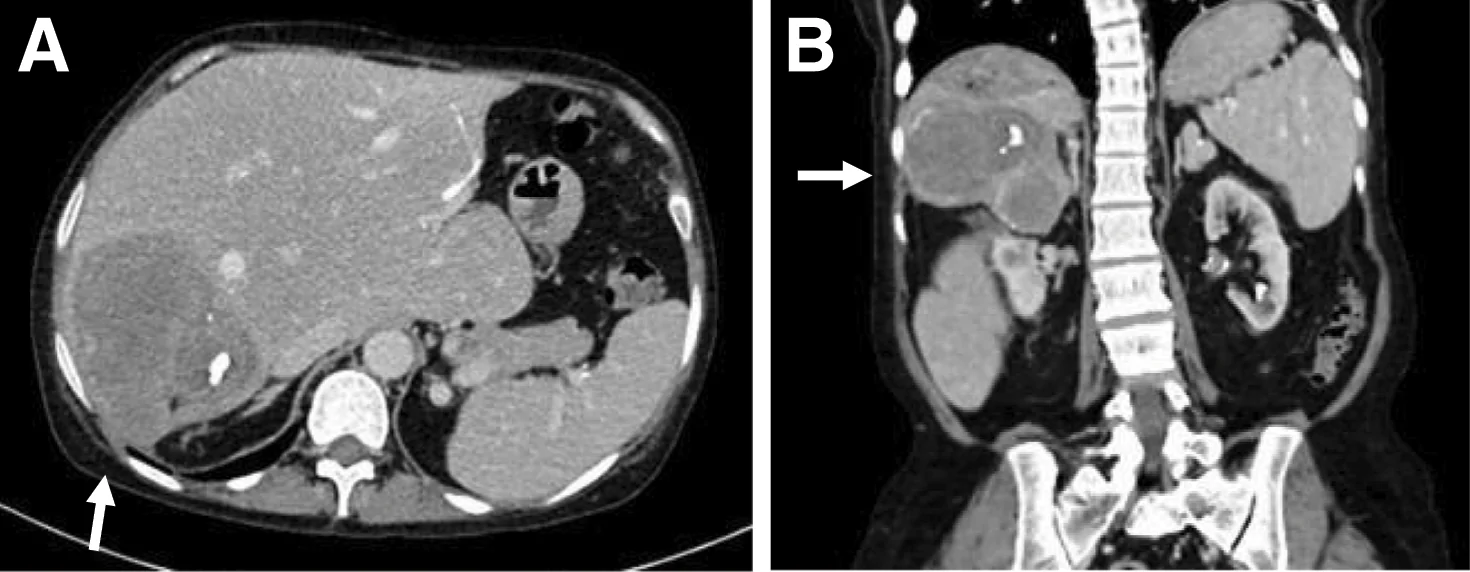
The CT findings of the tumor-like lesions containing calcifications and fat observed in the S7 region of the liver before the SLKT (A and B). SLKT, simultaneous liver-kidney transplantation
